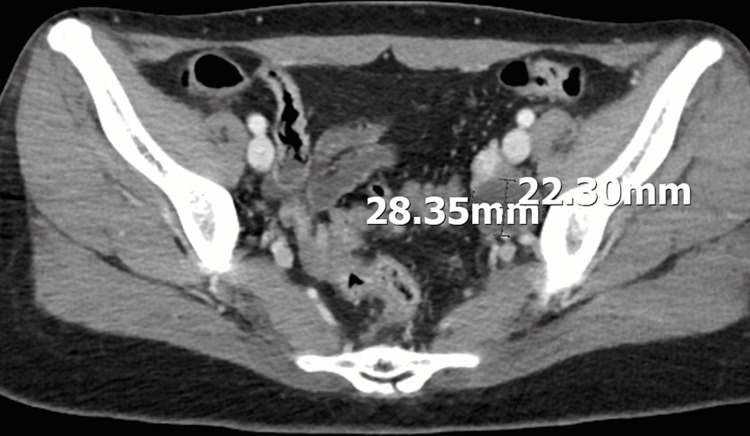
Pelvic CT showed a tumor that looked like a functional left ovarian cyst, which measured about 2.8 cm.
